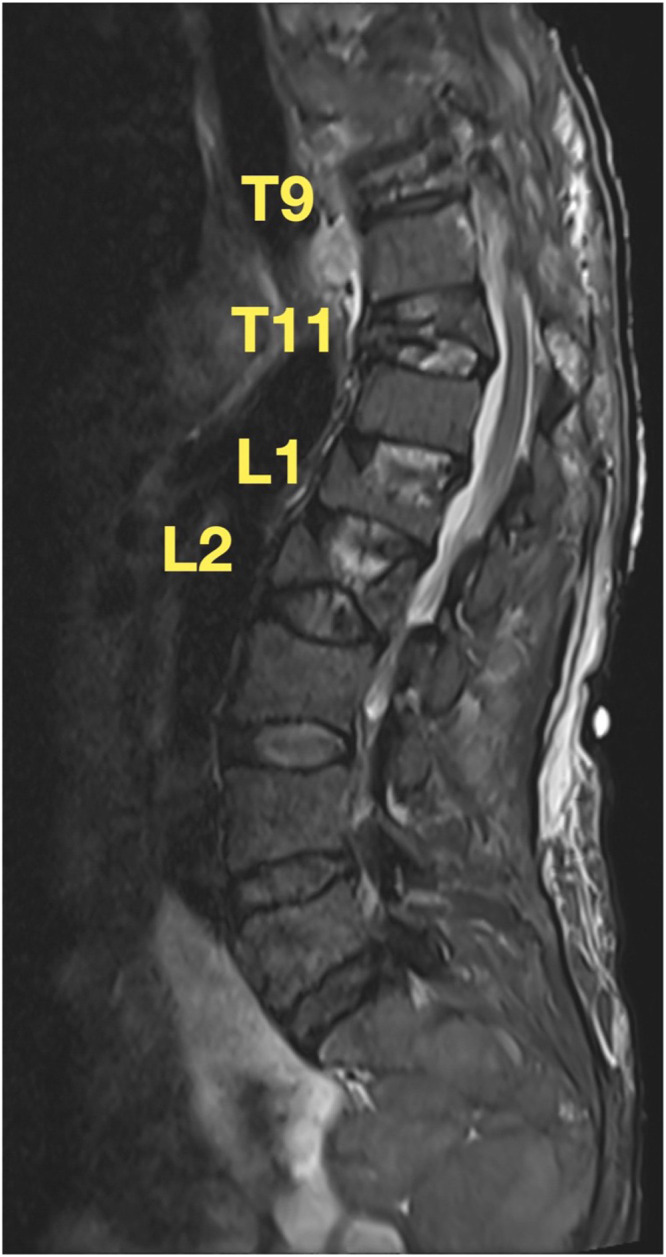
Lumbar MRI at 6-weeks postoperatively demonstrating postsurgical changes with no recurrence of dorsal extradural masses.
